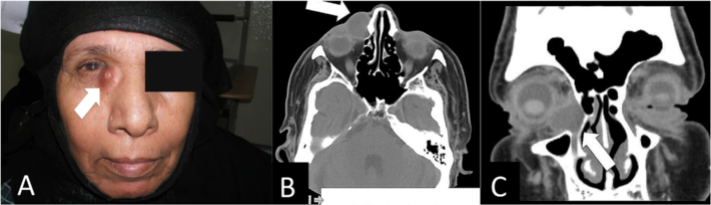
Preoperative diagnosis. a A Clinical photo of a patient with right eye dacrocele. b CT Axial cut showing medial canthus dacrocele. c CT Coronal cut showing the same finding
